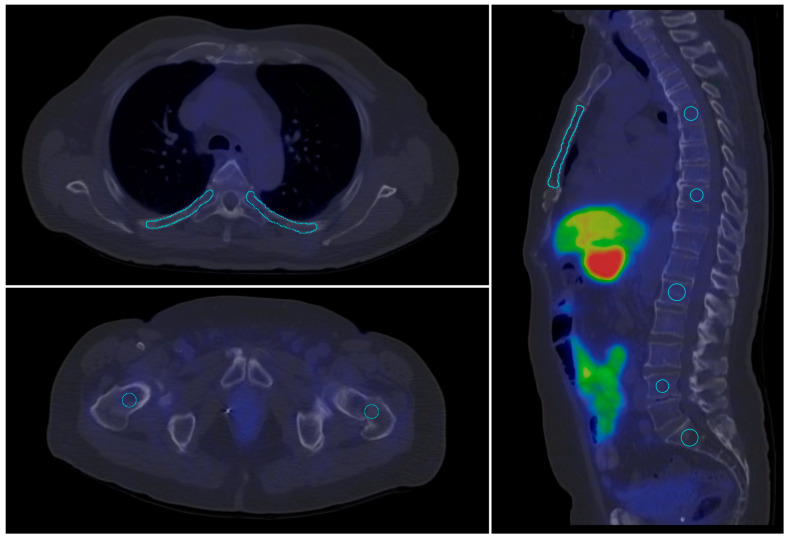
Contoured skeletal volumes of interest (VOI) (blue lines) in standard locations on a [^18^F]F-JK-PSMA PET/CT scan.
